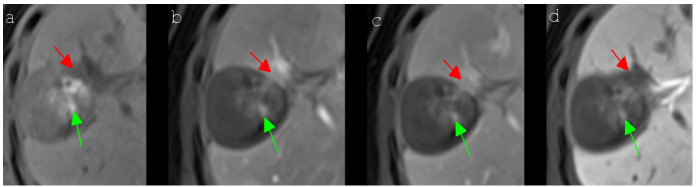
A 64-year-old woman with a local tumor progression in the peripheral ablation zone (segment V/VIII) six months after IRE of an HCC, which presented a typical signal behavior in Gd-EOB-DTPA-based MR imaging. The HCC showed a hypointense signal (red arrow) in the unenhanced T1 MR image (a), an obvious hyperintense signal (red arrow) in the contrast-enhanced T1 arterial phase MR image (b), a slight wash-out (red arrow) in the contrast-enhanced T1 portal venous phase MR image (c) and a hypointense signal (red arrow) in the contrast-enhanced T1 delayed phase MR image (d). In addition to this, the central part of the ablation area showed several hyperintense spots (green arrow), which could be detected in all presented MRI sequences (a–d), being caused by post-interventional bleeding.
